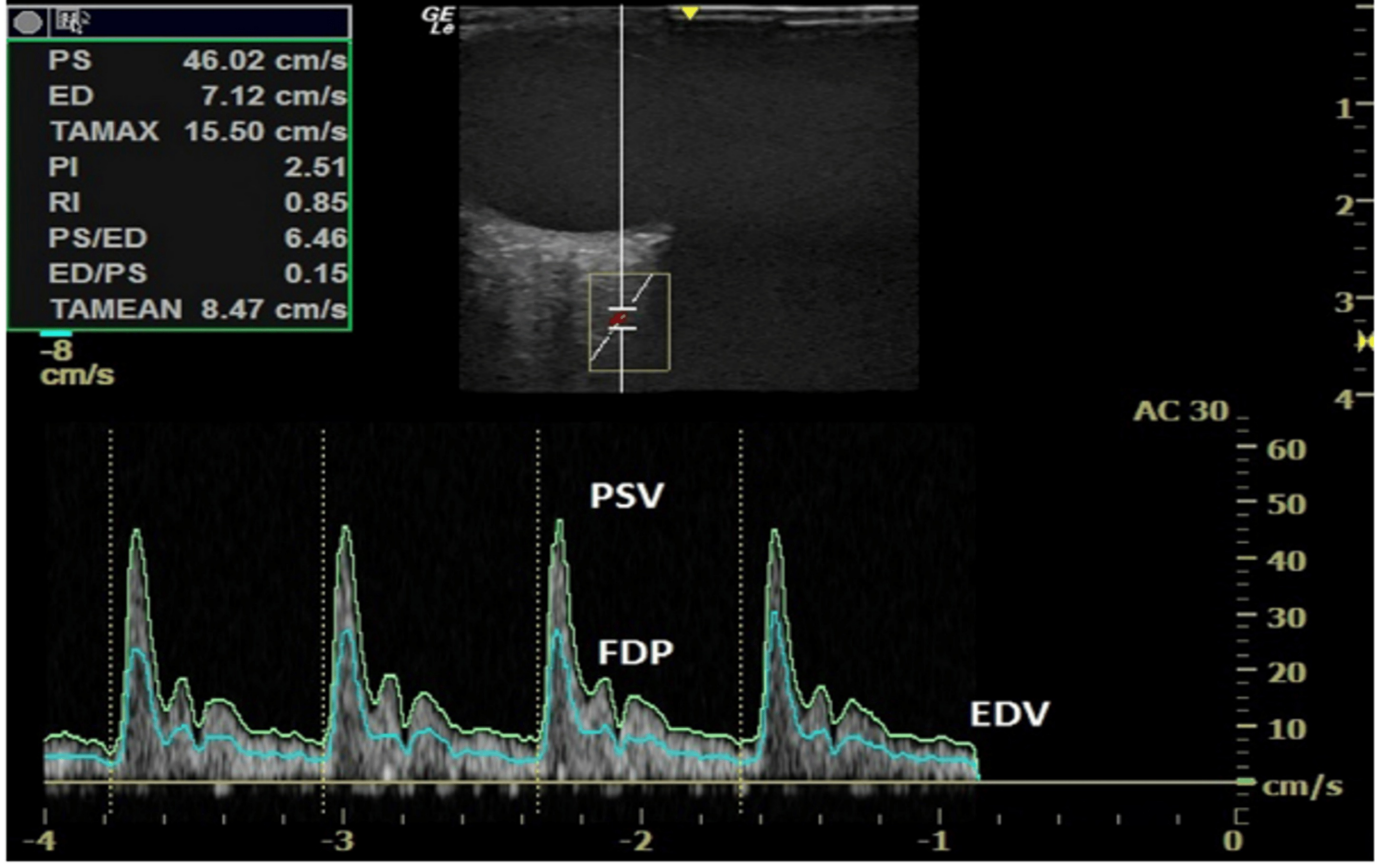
B-mode ultrasound image of orbit and retro-orbital structures, with superimposed color Doppler for identification of the ophthalmic artery, and pulsed-wave Doppler to acquire its spectral waveform. EDV: End-diastolic velocity; FDP: first diastolic peak velocity; PSV: peak systolic velocity.
